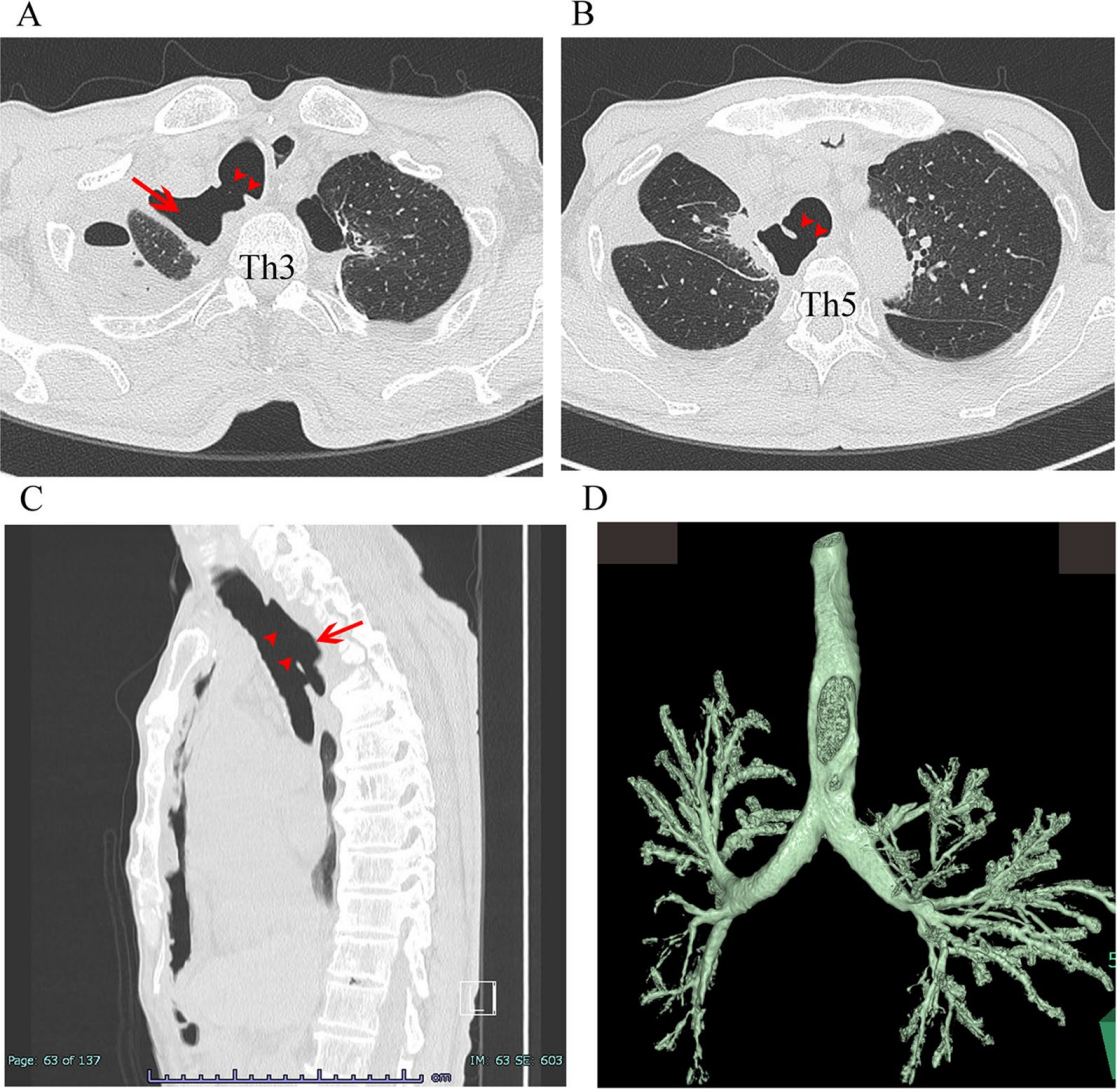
Initial chest CT findings of tracheal necrosis. Axial view at Th3 level (A) and Th5 level (B), sagittal view (C), and 3D-CT reconstruction image (D). The posterior wall of the trachea was missing an area of 16 × 42 mm (arrowheads) and connected to the dead space (arrow). *Th* thoracic vertebra, *CT* computed tomography
